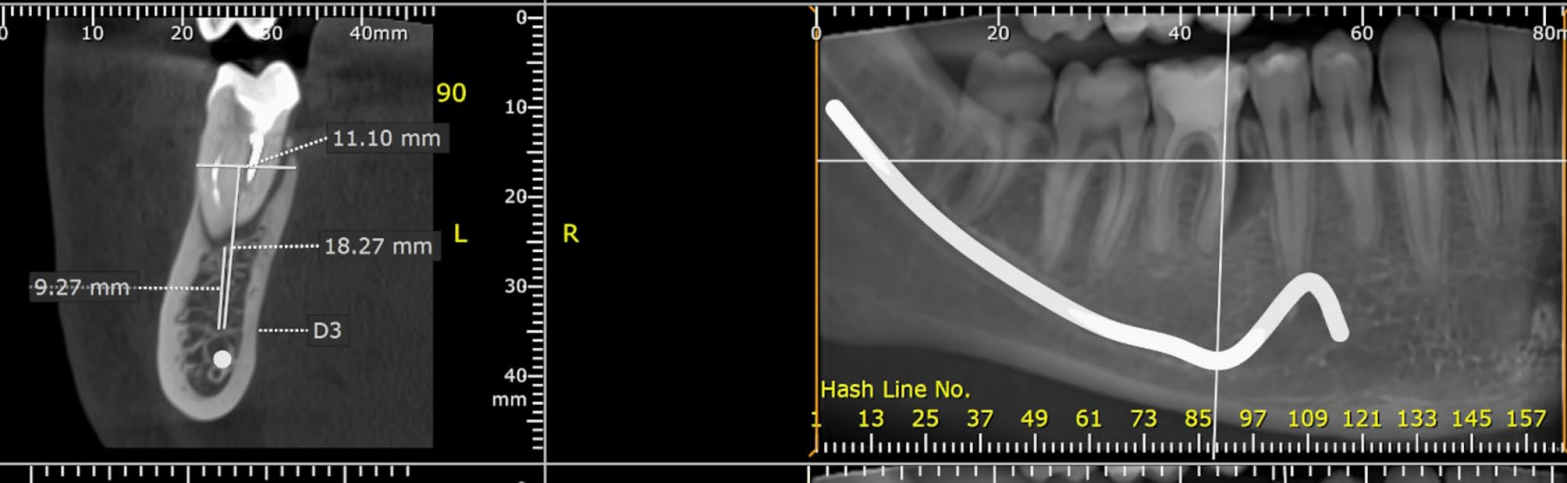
Preoperative CBCT for non-restorable lower left first molar. Note the absence of buccal bone
